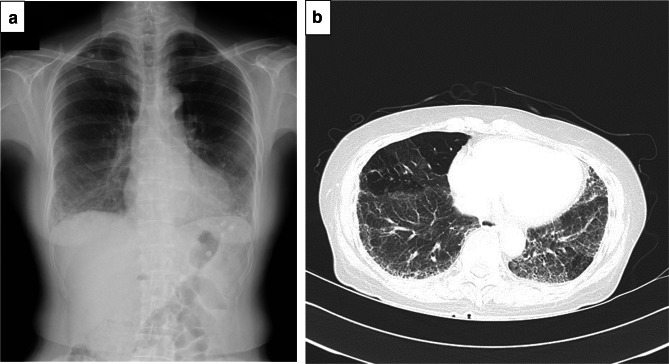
Preoperative pulmonary findings. a chest radiograph on admission showed ground-glass opacities in the bilateral lower lung fields. b chest CT showed ground-glass opacities and reticular shadows in the bilateral lung fields
